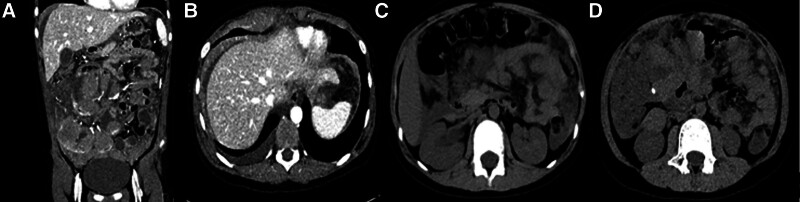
Abdominal CT: There were 2 oval high-density lesions in the small intestine in the abdomen (Se6/Img130, 193), the larger one was about 1.0 cm × 0.4 cm (B, D). There was no abnormal density shadow and abnormal enhancement focus in the remaining abdominal intestine. In the lower abdomen, the peritoneum, omentum and mesentery were thickened with blurred edges (A, C). Multiple lymph nodes were seen in the mesentery area, and the short diameter of the larger one was about 1.0 cm. CT = computerized tomography.
